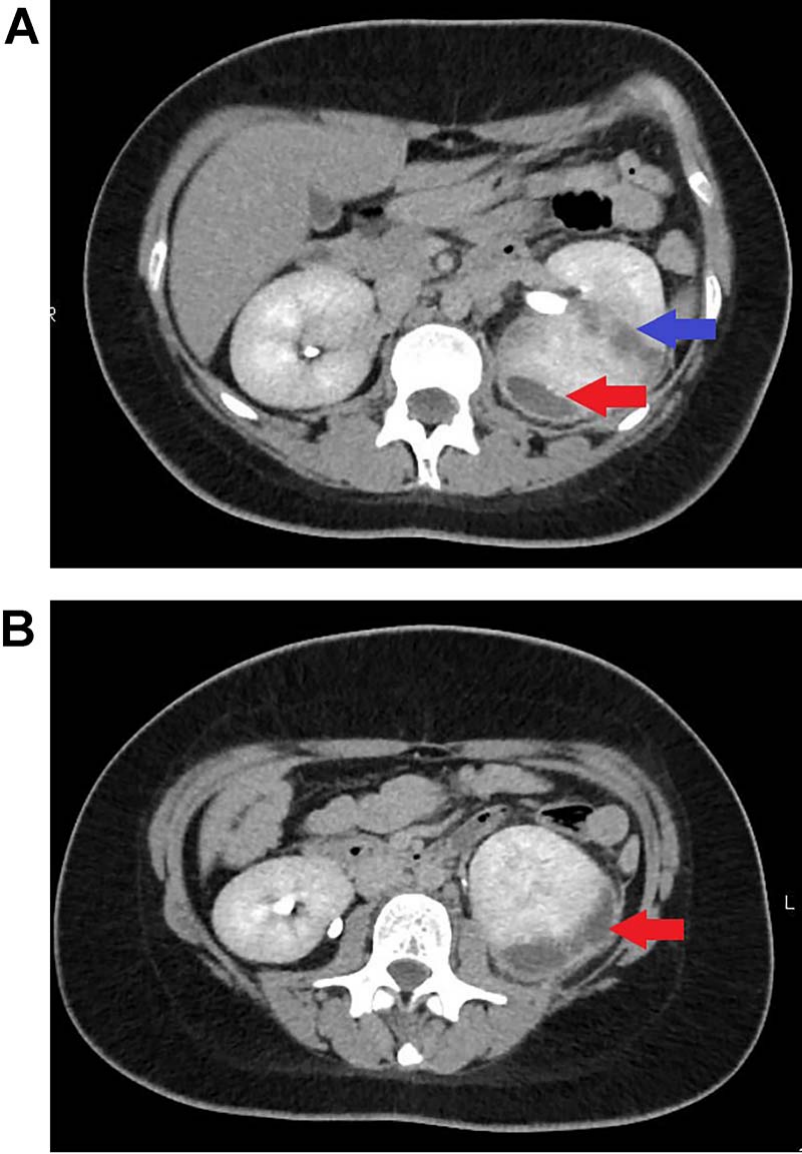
(a) Renal CT scan showing an abscess in the left kidney (blue arrow) and subcapsular perinephric collection (red arrow). (b) Renal CT scan showing subcapsular perinephric collection in the left kidney (red arrow).
